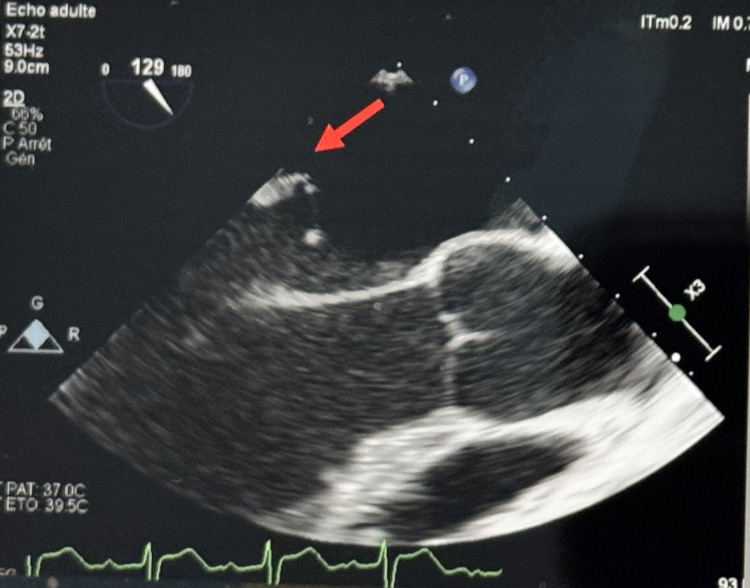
Transoesophageal echocardiography 3 chamber view showing a mobile, filiform structure measuring 17 mm attached to the atrial side of the P2 scallop of the mitral valve
